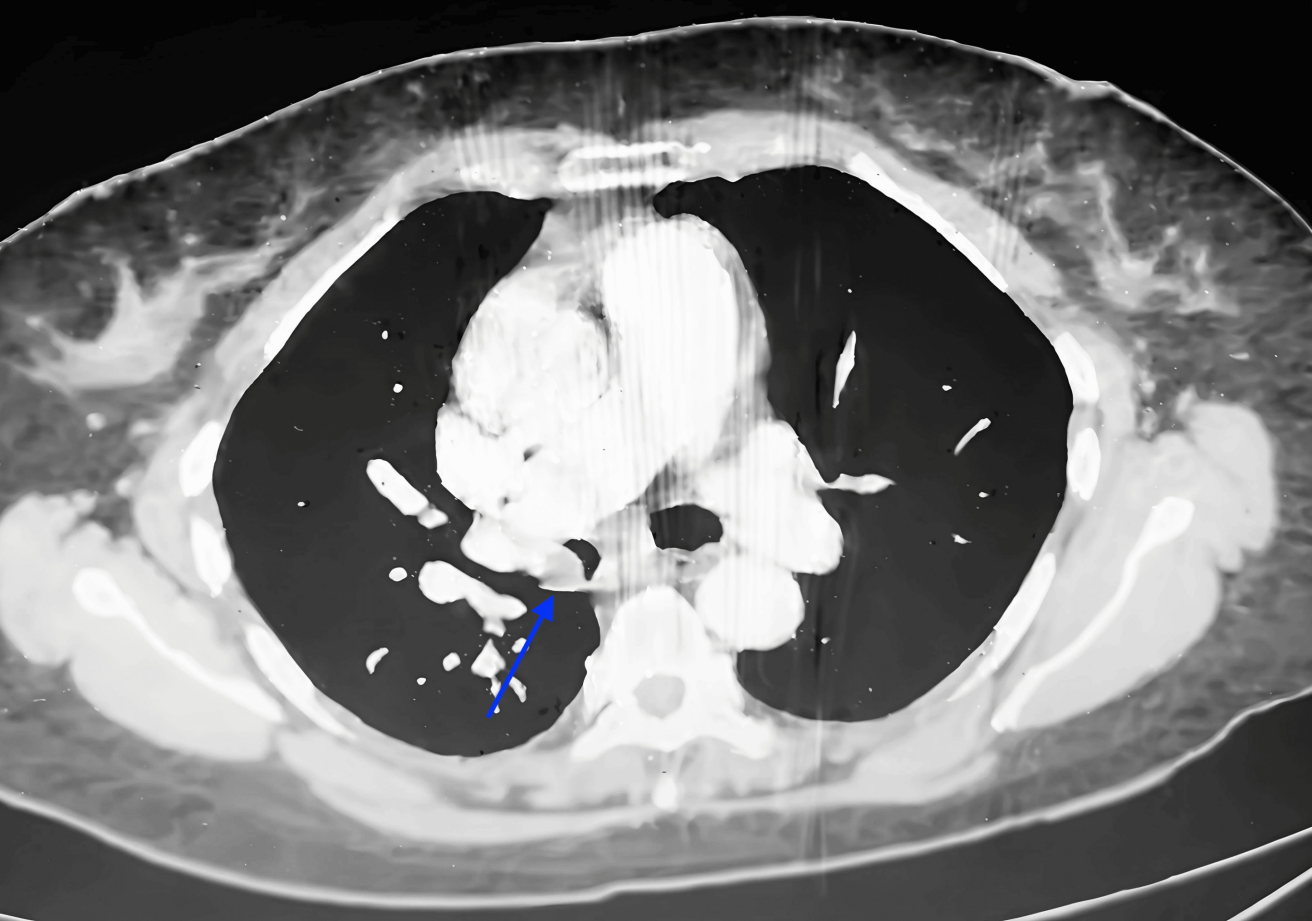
Axial CT of the thorax (mediastinal window) reveals an endobronchial obstruction in the right main bronchus Axial CT of the thorax (mediastinal window) demonstrates a well-defined lesion (14 × 18 mm) (blue arrow) within the right main bronchus, causing near-complete endobronchial obstruction. CT, Computed Tomography
